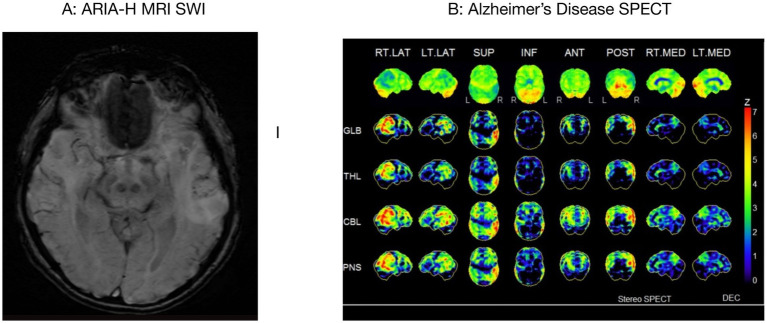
(A) ARIA-H MRI SWI. (B) Alzheimer’s disease SPECT.
